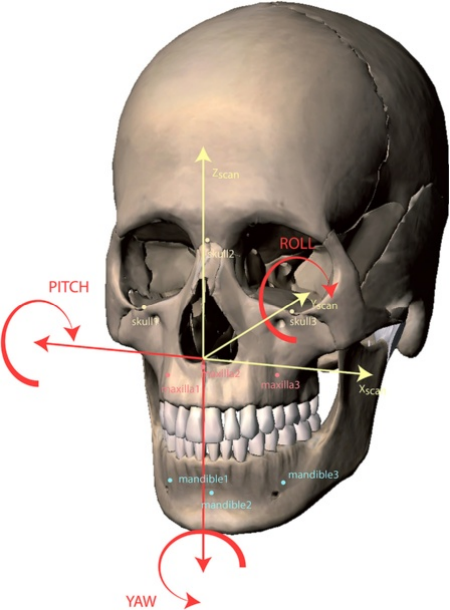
3D CT scan image view showing the rotational (pitch, roll and yaw) movements measuredThree vectors on the planned pre- (V_plan1_;V_plan2_;V_plan3_) and post-operative (V_ppop1_;V_ppop2_;V_ppop3_) were then determined to describe the space of the three anatomical regions (Fig. 7):
